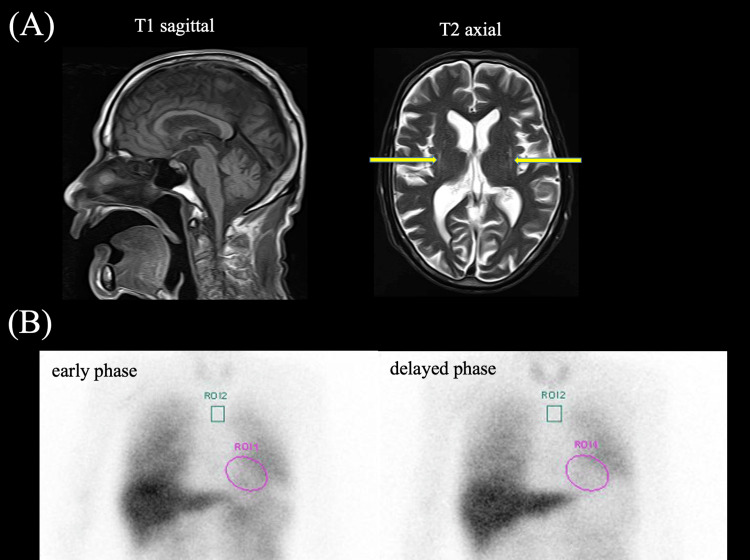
Follow-up imaging findings and changes in the cardiac sympathetic function (A) Follow-up brain magnetic resonance imaging showed hyperintense rims along the lateral margins of the bilateral putamina on T2-weighted imaging (yellow arrows), suggestive of multiple system atrophy–parkinsonian type (MSA-P); (B) After selegiline discontinuation for 28 days, repeat cardiac ^123^I-metaiodobenzylguanidine scintigraphy showed improved early and delayed H/M ratios of 2.653 and 2.032, respectively, with a washout rate of 46.0%. The institutional reference values were early and delayed H/M ratios >2.0 and a washout rate <34%.
